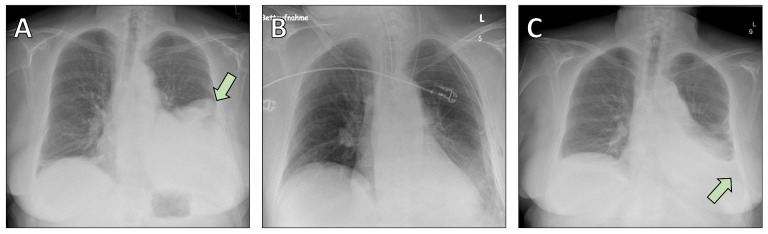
Chest radiographs obtained during the perioperative period. (A) Preoperative chest X-ray showing a large opacity corresponding to the solitary fibrous tumor in the left lower lobe (arrow). (B) Postoperative chest X-ray demonstrating satisfactory re-expansion of the remaining lung parenchyma following left lower lobectomy. (C) Postoperative image revealing a pleural effusion (arrow), which was successfully managed with conservative treatment using diuretics.
